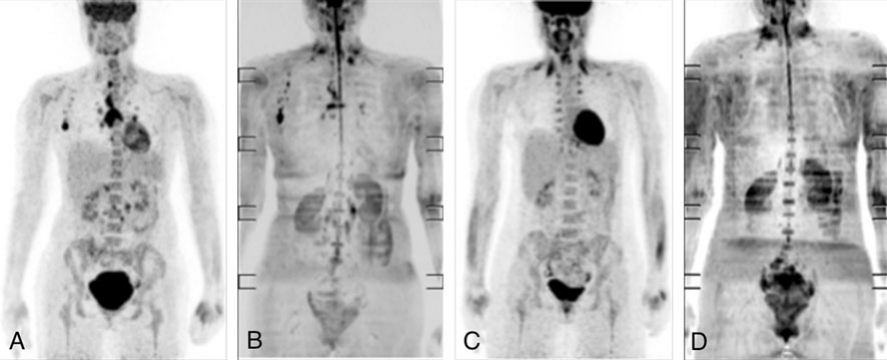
FDG-PET/MR in a patient with Hodgkin’s disease before and after chemotherapy. a PET MIP image showing enlarged lymph nodes with increased FDG uptake in the mediastinum and the right axilla. b Inverted MIP of diffusion weighted MR images acquired during the same exam, with the lymphoma mentioned above clearly recognizable. c PET MIP after chemotherapy, showing complete response. d Corresponding inverted DWIBS MIP. Non-specific symmetric FDG-accumulation in brown fat tissue in the lower cervical and supraclavicular region on both PET scans (more pronounced on the second PET with additional paravertebral symmetric FDG uptake) should not be mistaken as lymphoma tissue. Signal decrease in the lower cervical region on the inverted diffusion weighted images is caused by inhomogenous fat suppression (DWIBS Images are acquired with the integrated body coil of the MR system)
